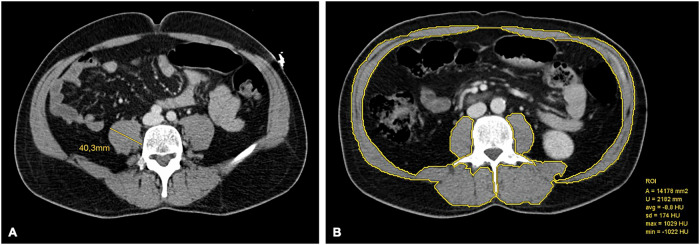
Measurement of skeletal muscle parameters. A Measurement of the transverse psoas muscle thickness perpendicular to the largest diameter of the psoas muscle on an axial, contrast-enhanced CT scan at the level of the umbilicus. B Measurement of the skeletal muscle area (SMA, cm^2^) on an axial, contrast-enhanced CT scan at the level of the L3 with transverse processes imaged. A, area; avg, average; HU, Hounsfield unit; max, maximum; min, minimum; sd, standard deviation, U, circumference
